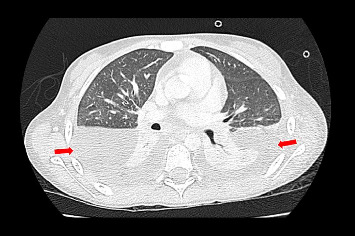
Findings from the CT scan performed during ICU stay. Extensive peripheral hypodensity was observed in the pulmonary parenchyma of both hemithoraces, corresponding with the bilateral pleural effusion indicated by the red arrows.
